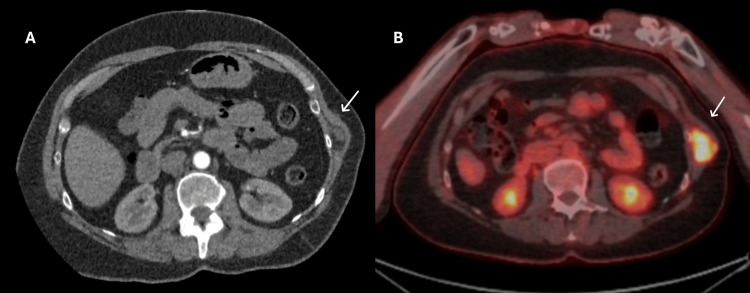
A: CT of the thorax and B: PET-CT A: CT of the thorax showing a well-defined, hypervascular mass on the left chest wall arising from the intercostal space of the 10th rib (arrow). B: PET-CT demonstrating a 3-cm left chest wall lesion with increased metabolic activity (SUVmax 13.1), consistent with a hypervascular soft tissue tumour (arrow).
